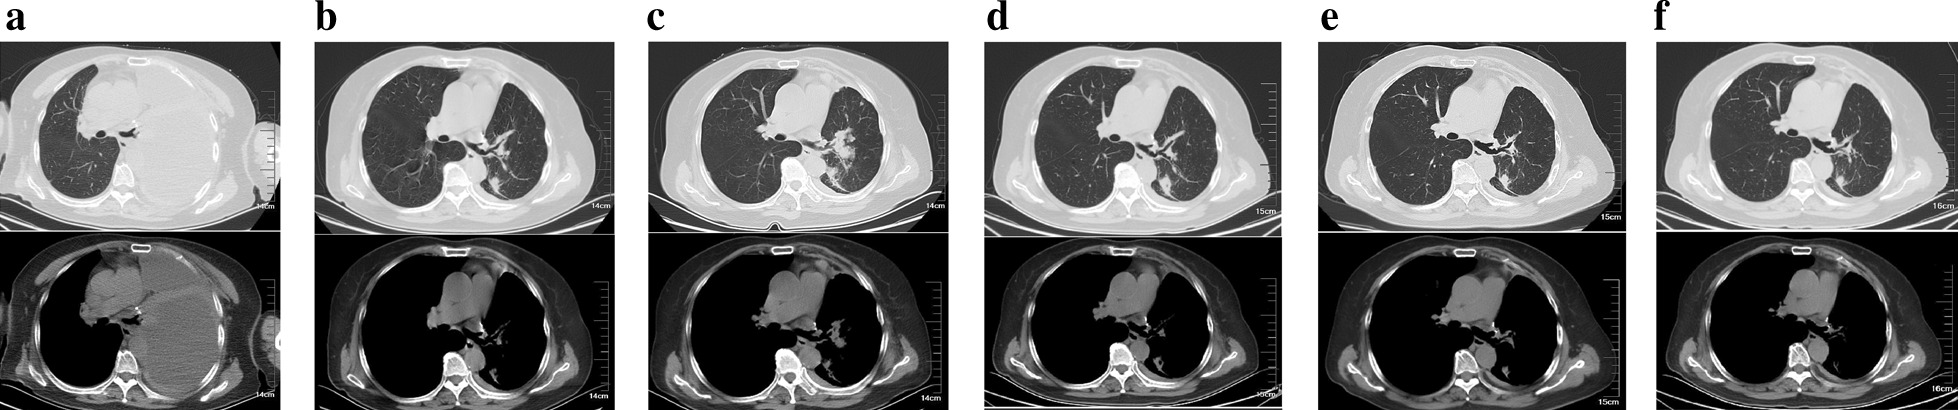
The chest computed tomography (CT) scan images showing the locations of tumor lesions found in a left pleural effusion of lung when the patient presented with dyspnea in June 2019; b after thoracentesis, two metastatic nodules in the left lower lobe; c after accepted PD-1 inhibitor Camrelizumab, 200 mg/time, twice per week, for a total of 4 injections (2 cycles), the tumor nodules were still increased, indicating the disease progressed; d After taking larotectinib, 200 mg/day, orally for 1 month, some lesions showed decreased in size; e oral administration of larotectinib for 3 months indicated some cancerous lesions were continuously reduced in size; f Oral administration of larotectinib for 6 months showed that some cancerous lesions either are reducing or even disappeared
